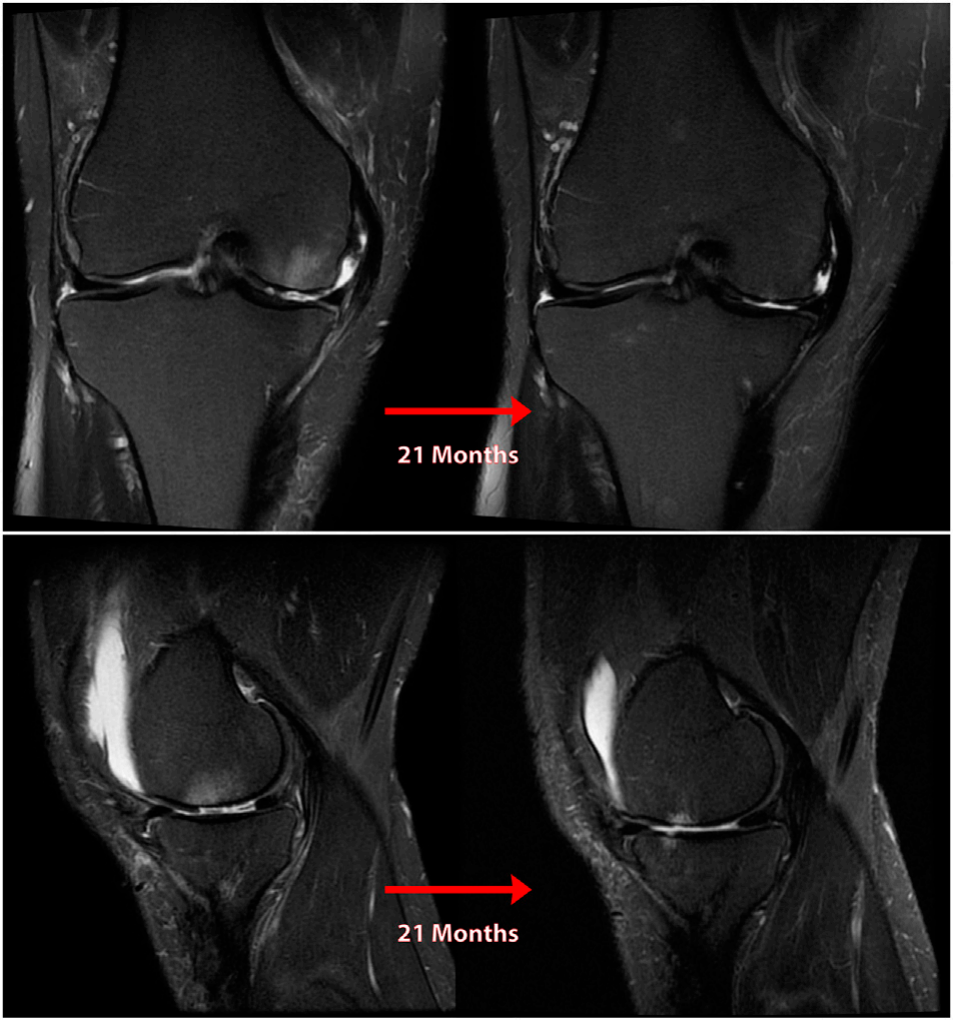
Coronal (A) and sagittal (B) MR images of nonanatomic root repair patient #5 showing progression of osteoarthritis in the medial compartment of the knee over 21 months secondary to nonanatomic medial meniscus root repair (right knee). M = medial; L = lateral; A = anterior; P = Posterior.
